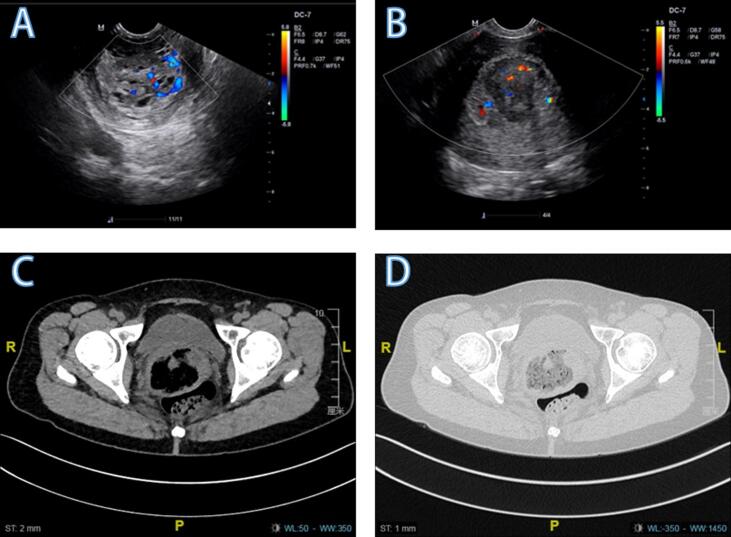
Pelvic ultrasound (A and B) and CT (C and D) showing a soft tissue mass protruding from the uterine cavity to the cervical area.
